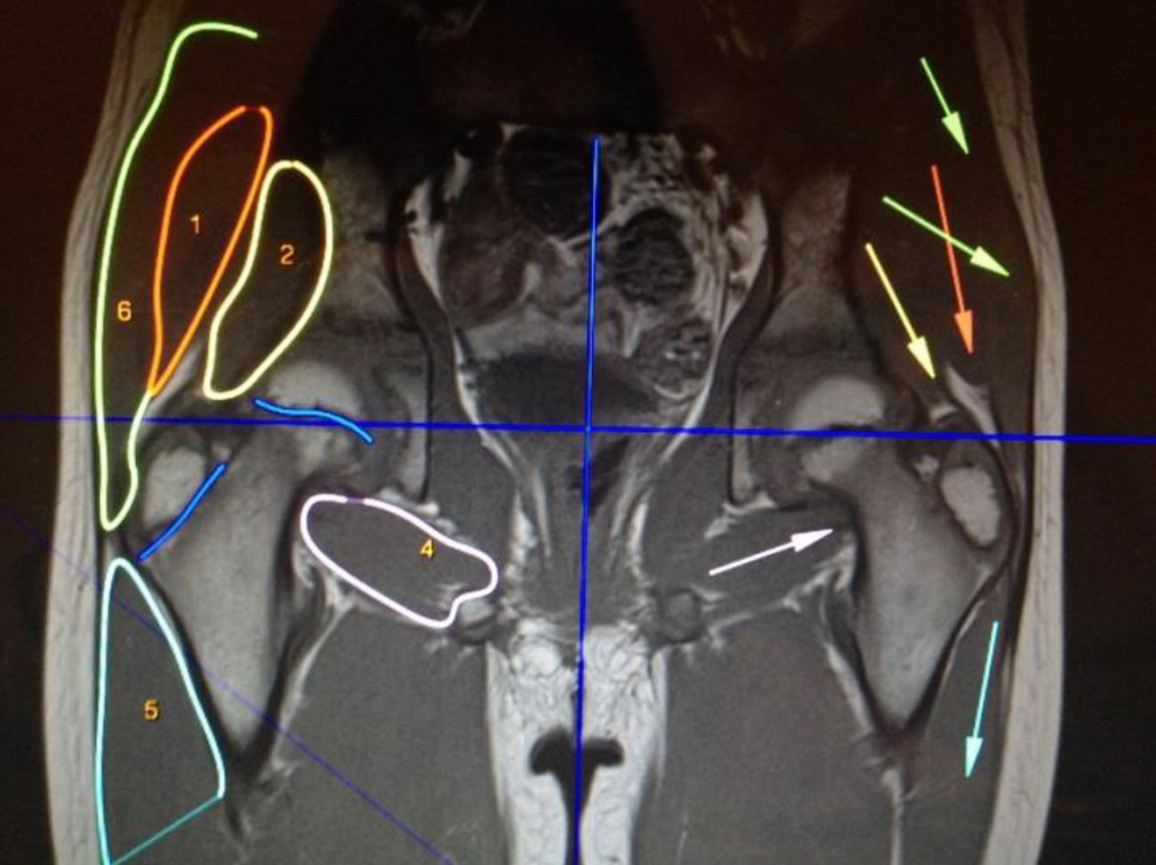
Frontal T1‐MRI with outlines (patient right) and straight lines (patient left). 1 Gluteus medius; 2 gluteus minimus; 4 quadratus femoris; 5 vastus lateralis; 6 gluteus maximus.
